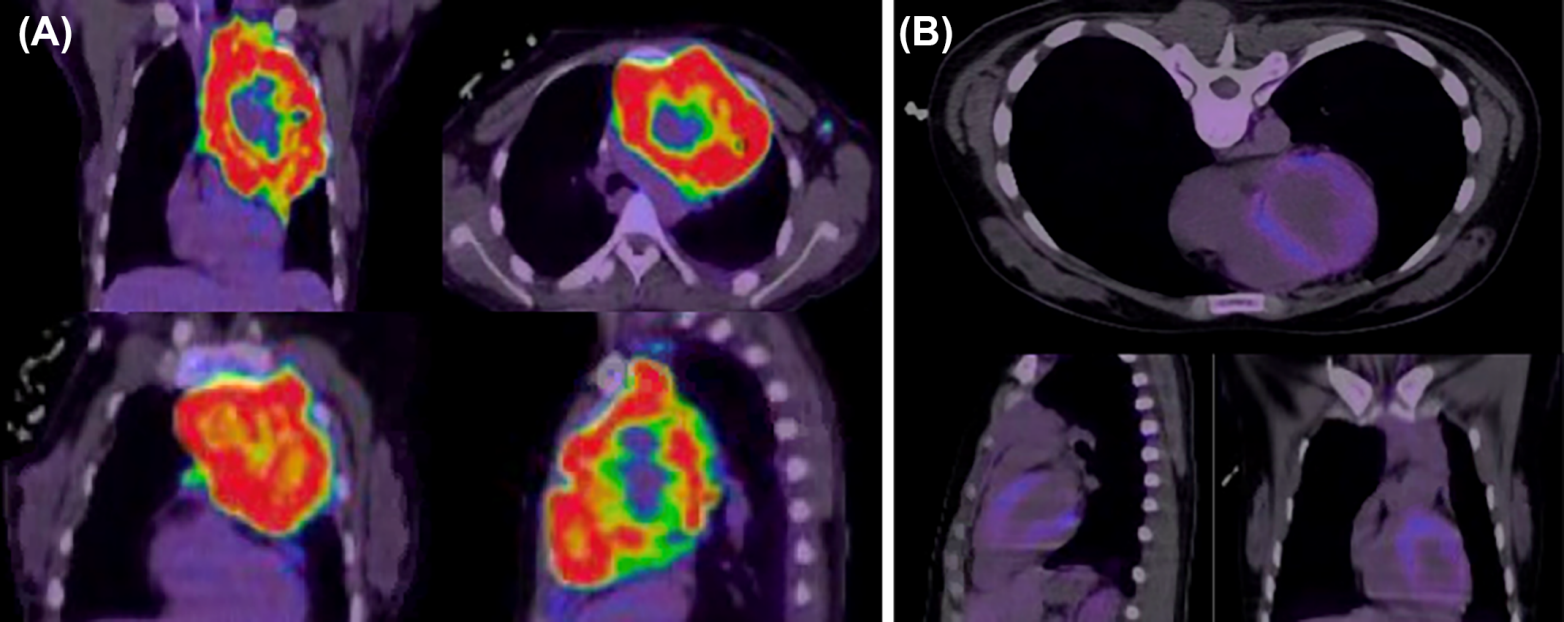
(A): NM FDG PET CT scan showing mediastinal mass consistent with lymphoma. (B): NM FDG PET CT scan showing mediastinal mass consistent with near complete metabolic resolution of the previously noted anterior mediastinal FDG avid mass lesion.
